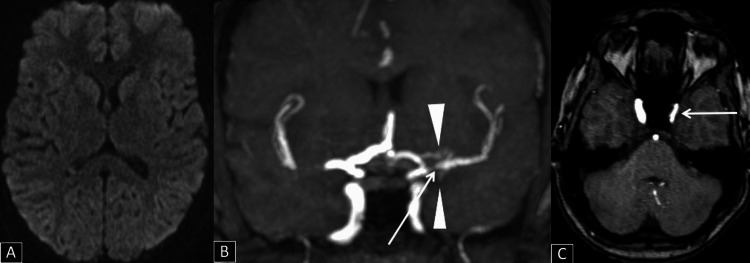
(A) Axial DWI showing no areas of diffusion restriction; (B) Reformatted coronal TOF MRA showing short segment tight stenosis (arrow) in the M1 segment of the left MCA, with few surrounding collaterals (arrowheads) in left MCA cistern; (C) Axial TOF MRA  showing moderate stenosis involving the cavernous segments of the left internal carotid artery (arrow). DWI: Diffusion-weighted image, TOF: Time of flight, MRA: Magnetic resonance angiography, MCA: Middle cerebral artery
